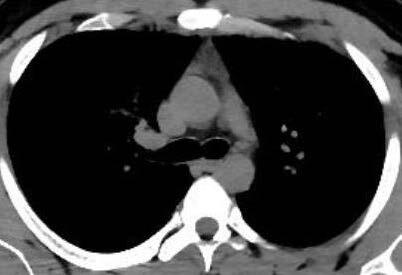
Axial thorax CT images in which the degree of thymus atenuation is assessed. Grade 3. Complete fatty degeneration of the thymus. b. Axial thorax CT images in which the degree of thymus atenuation is assessed. Grade 2. Thymus gland is mostly fatty and reticulonodular densities are observed. c. Axial thorax CT images in which the degree of thymus atenuation is assessed. Grade 1. The thymus is triangular in shape and consists of half solid and half fatty tissue. d. Axial thorax CT images in which the degree of thymus atenuation is assessed. Grade 0. Solid thymus tissue consisting of the majority soft tissue.
